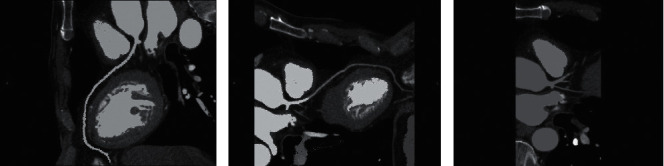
CT scan results of patients with coronary heart disease. (a) Patient 1, female, 58 years old, presented with left coronary artery stenosis and soft plaque; (b) patient 2, female, 56 years old, presented with calcification and stenosis of anterior descending branch, imbalance between blood supply and myocardial oxygen consumption, resulting in insufficient blood supply; (c) patient 3, male, 61 years old, presented with right coronary artery stenosis.
